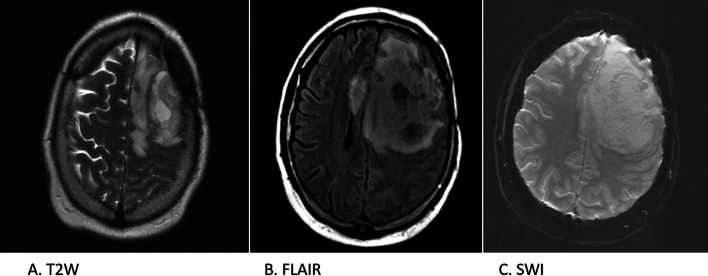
Case 2 brain MRI axial plane. Imaging shows lobulated heterogeneous solid left posterior frontal mass with foci of internal hemorrhage and surrounding edema causing significant mass effect and mild to moderate midline shift. A. T2WI = T2 weighted image. B. FLAIR = Fluid attenuated inversion recovery. C. SWI = Susceptibility weighted imaging
